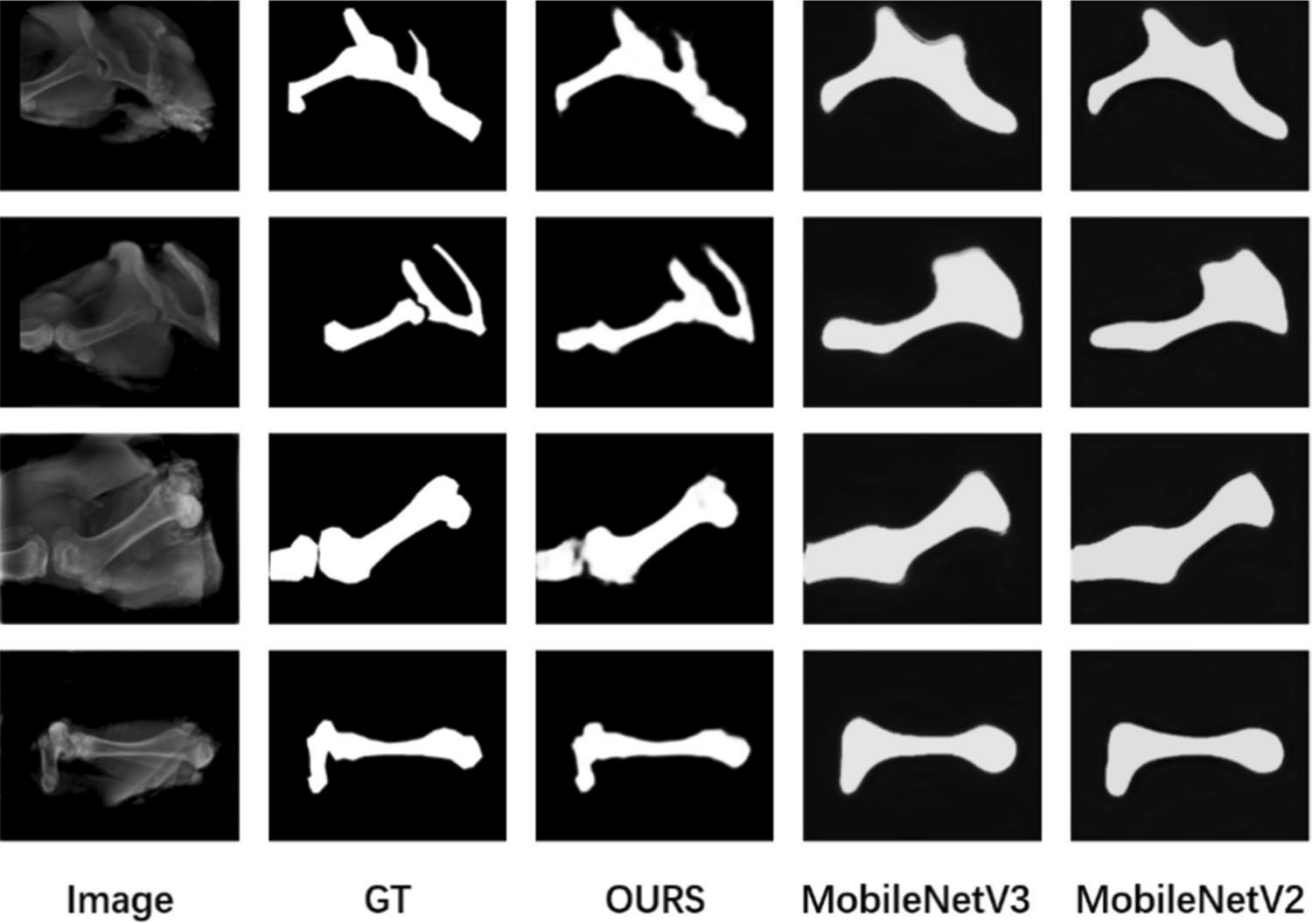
Qualitative comparison of the proposed method with a generic lightweight method on X-ray images.
